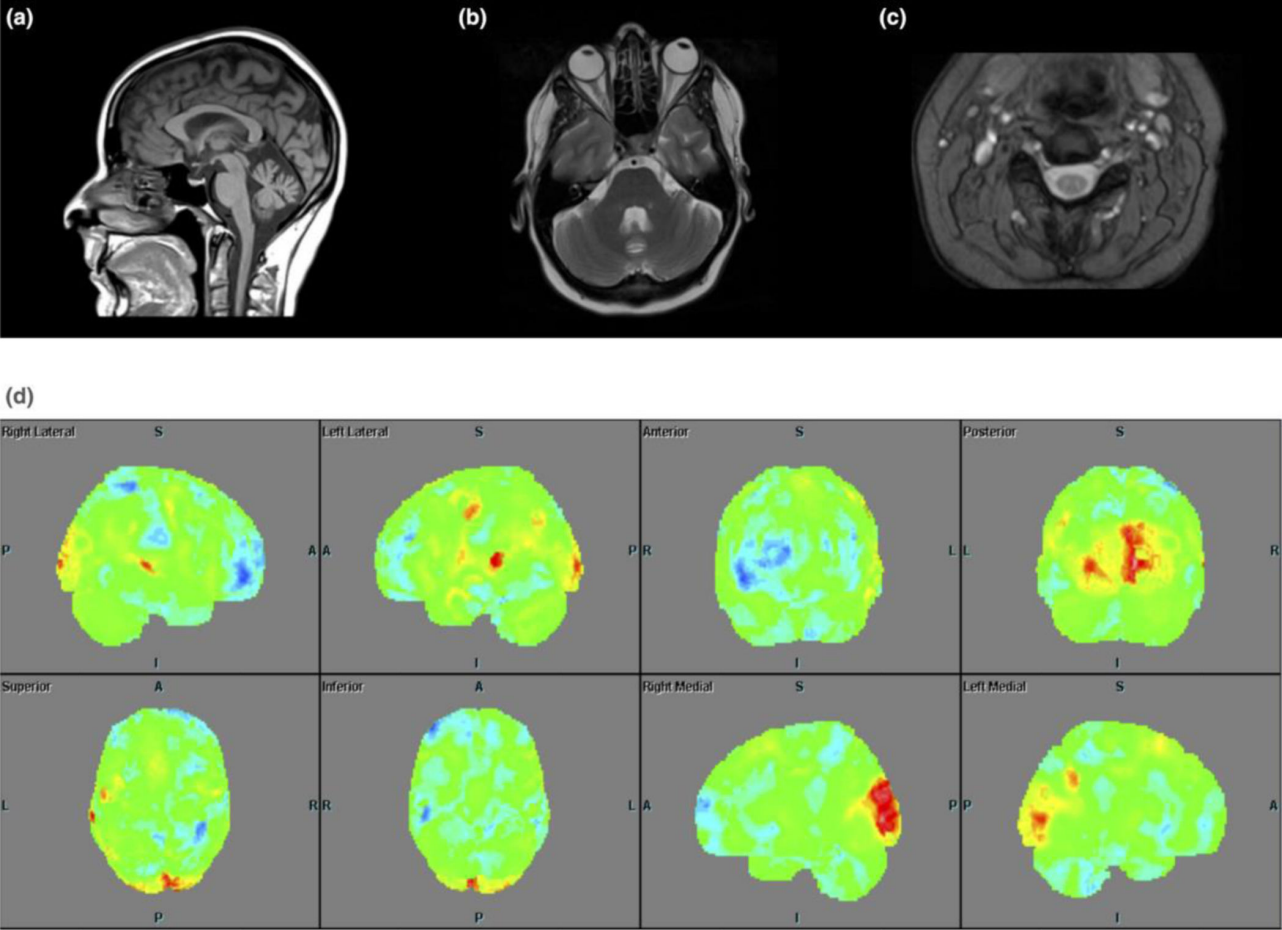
Brain and cervical MRI in patient III:2 at 34 years of age on a 1.5 T scanner showing mild to moderate vermal atrophy (a: mid-sagittal T1-weighted imaging, b: 4 mm thick slice; axial T2-weighted imaging, 5 mm thick slice) and mild atrophy of cervical spinal cord (c: T2*-weighted imaging at the C3-level, 4 mm thick slice). (d) Widespread moderate hypometabolism is evident on this 3D-SSP figure; the patient was not on any psychotropic medication. The affected areas are PFC, temporal cortex, putamen, vermis and pons. No shown is cingulate gyrus.
